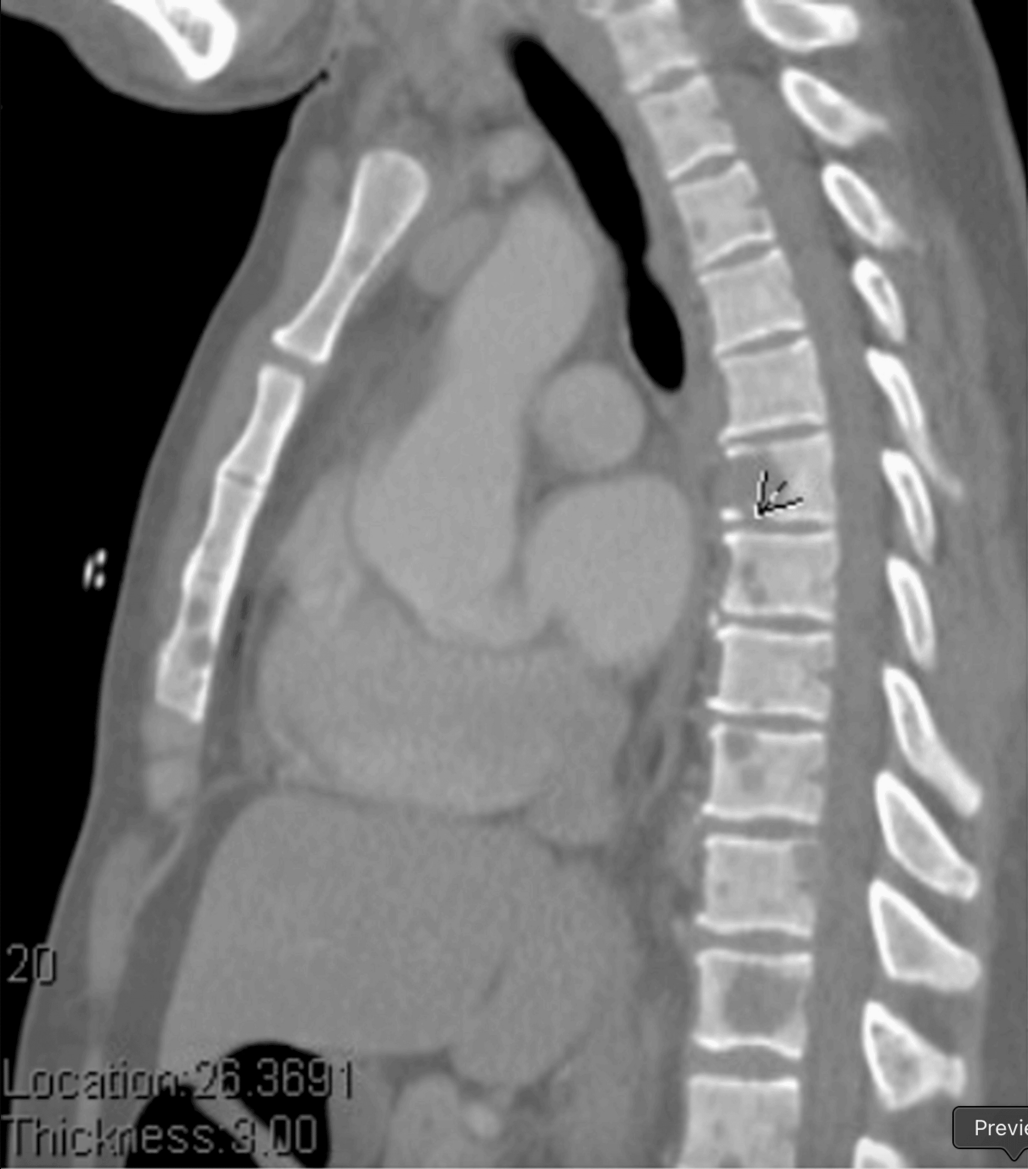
Computed tomography with contrast of the patient's chest The image reveals diffuse lytic lesions throughout the visible bones of the chest wall. One of the dominant lytic lesions at the T6 vertebral body (arrow) is associated with a small non-displaced fracture. Another large lytic lesion replaces much of the T11 vertebral body including the right posterior elements.
